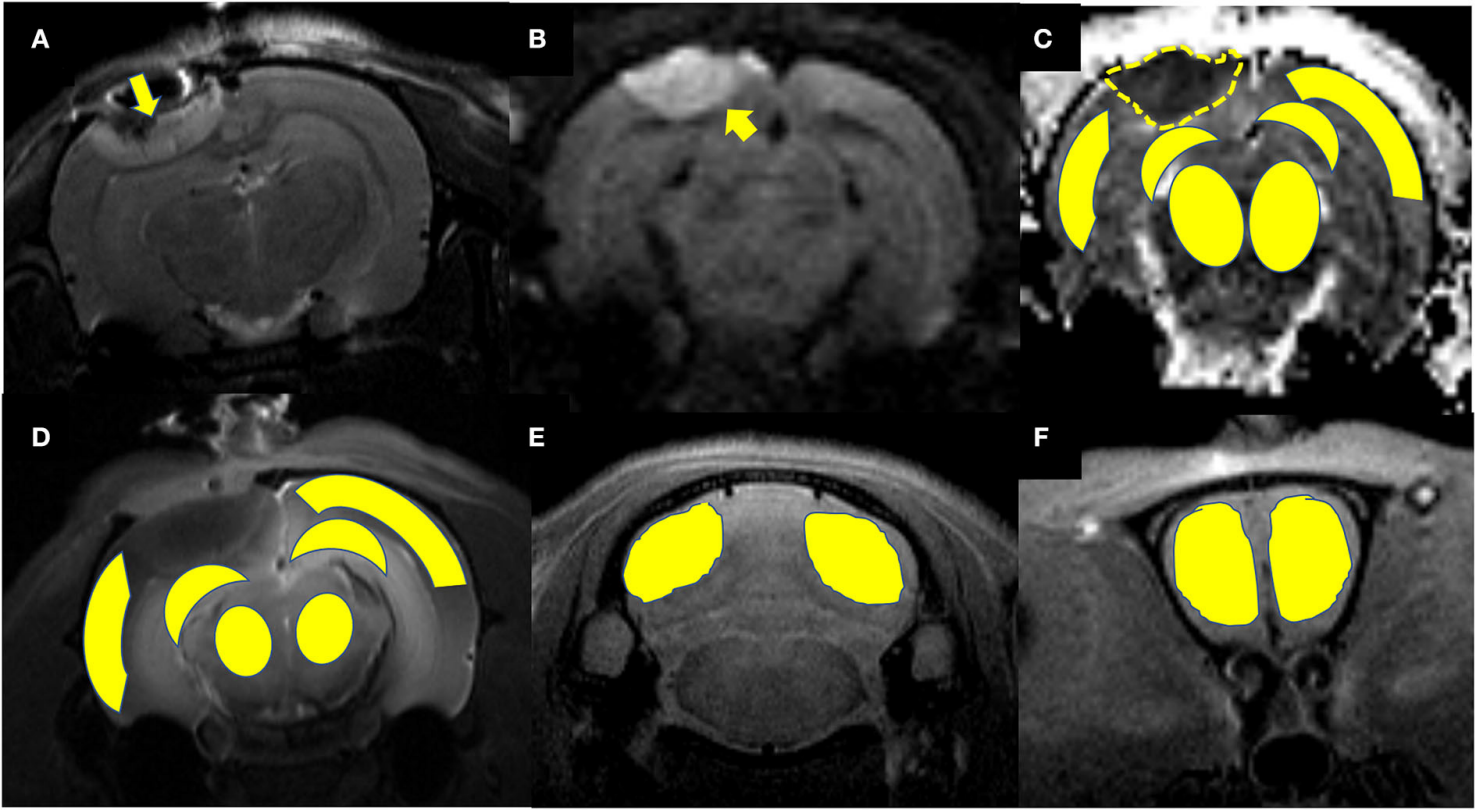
Representative images describing the creation of regions of interest (ROIs) for obtaining signal intensity (SI) changes before and after intrathecal contrast agent delivery and for obtaining the diffusivity of the brain subregions before and after temperature management. (A) Coronal T2WI and (B) axial DWI are used as a reference to direct the ROIs creation in the brain subregion on T1WIs and ADC maps. A traumatic lesion (yellow arrow) and bilateral cortex, hippocampus, and thalamus can be visualized. (C) Depicts the diffusivity of traumatic lesions and subregions obtained from the ADC map. The yellow dashed line defines the area of edema as marked manually by an independent observer. The area measurement on a series of slices was followed by calculating edema volume by defined slice thickness. (D) The signal intensity change after Magnevist delivery is obtained from the brain subregion, including bilateral cortex (ROI in injured site excludes the lesion), hippocampus, thalamus, and (E) cerebellum, and (F) olfactory bulb on coronal T1WI sections.
